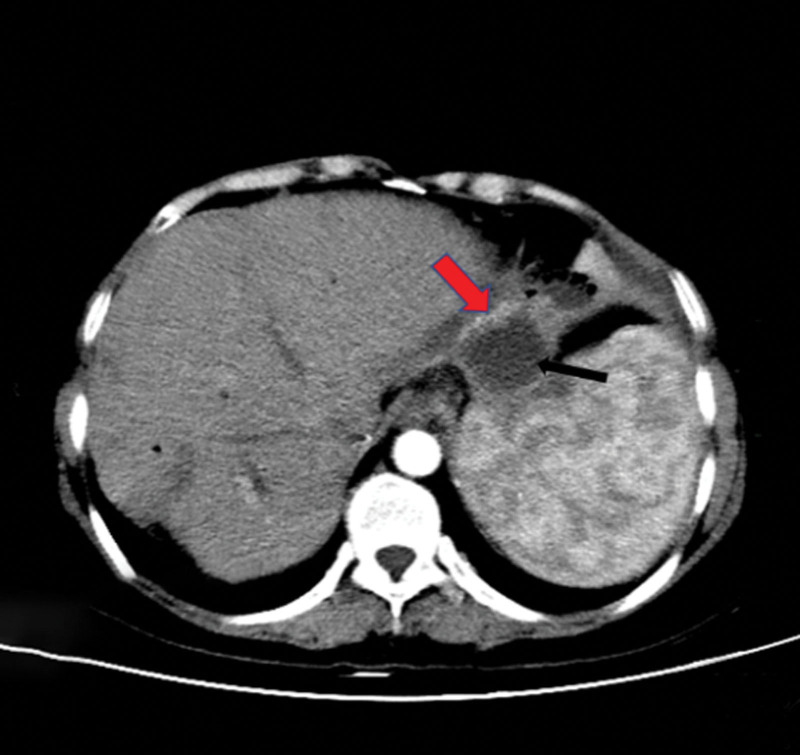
Enhanced CT image scans demonstrate a subphrenic abscess (Black arrow); the inferior phrenic artery passes from the front of the subphrenic abscess (red arrow). CT = computed tomography.
